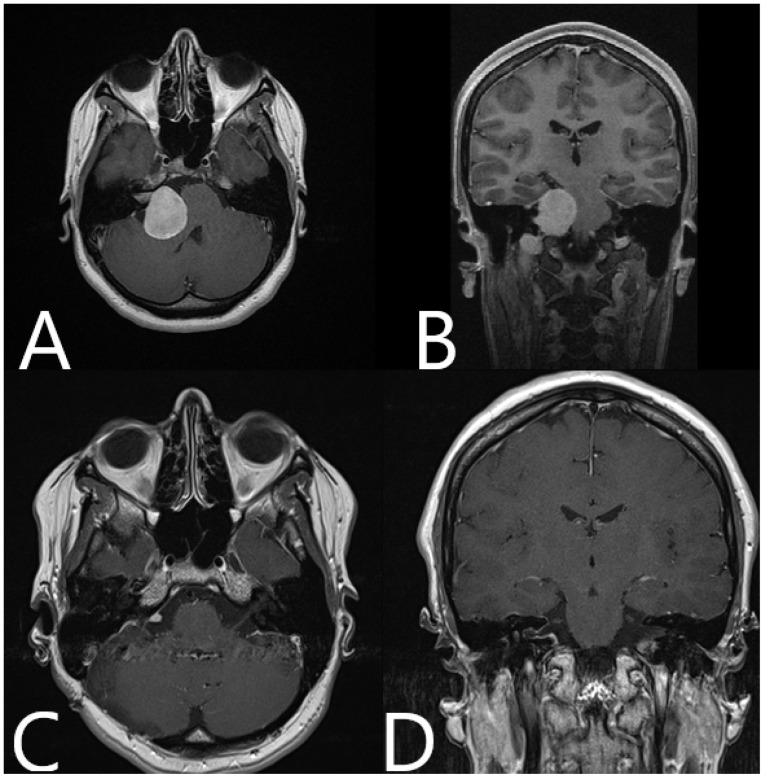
Patient No. 33. Preoperative (A) axial and (B) coronar T1-weighted post-contrast MRI with postoperative (C) axial and (D) coronar T1-weighted post-constrast MRI, depicting subtotal resection of the tumor and slight contrast enhancement along the course of the facial nerve.
